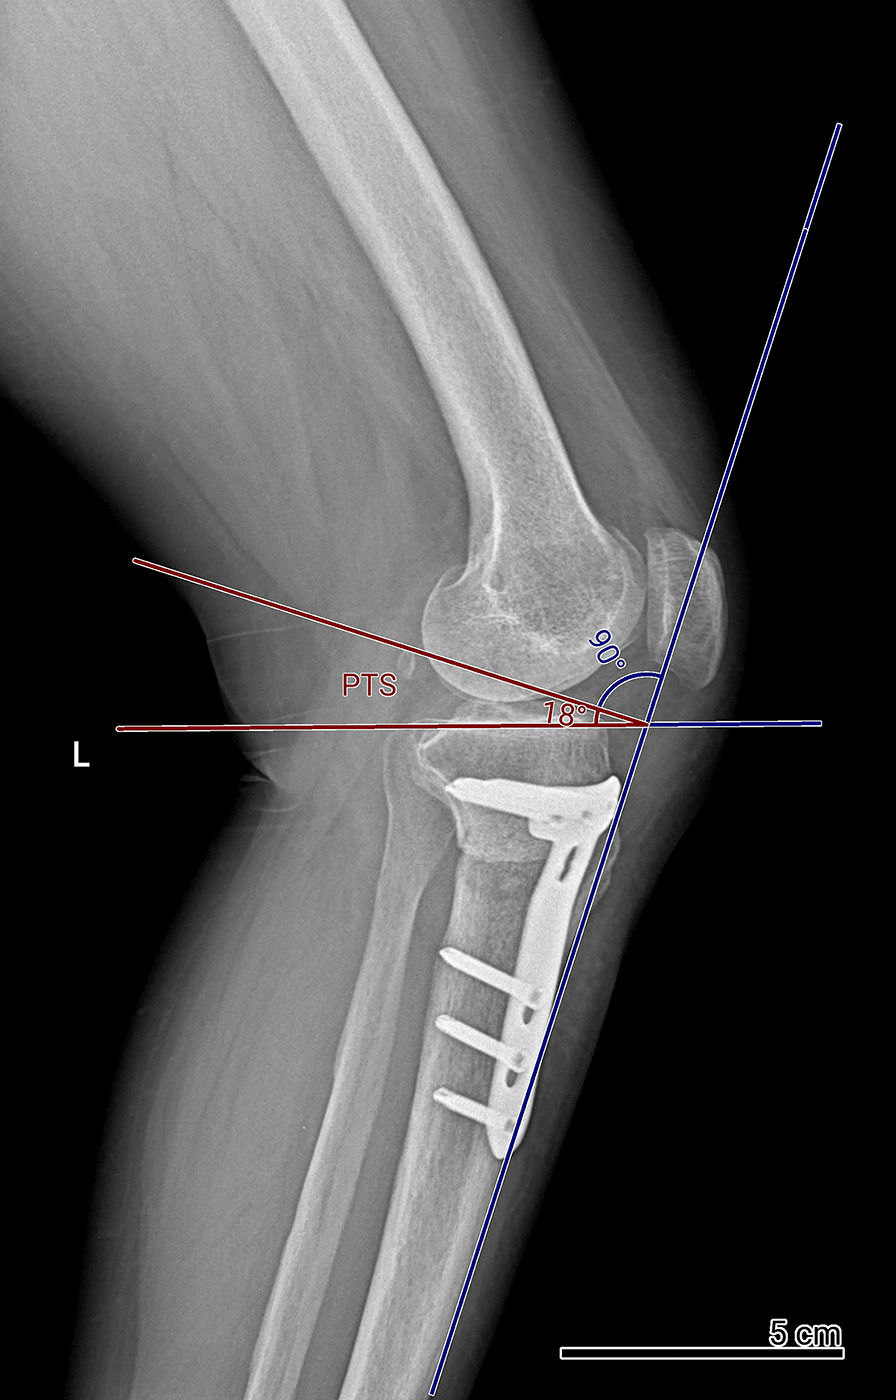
PTS measurement method in the radiograph
